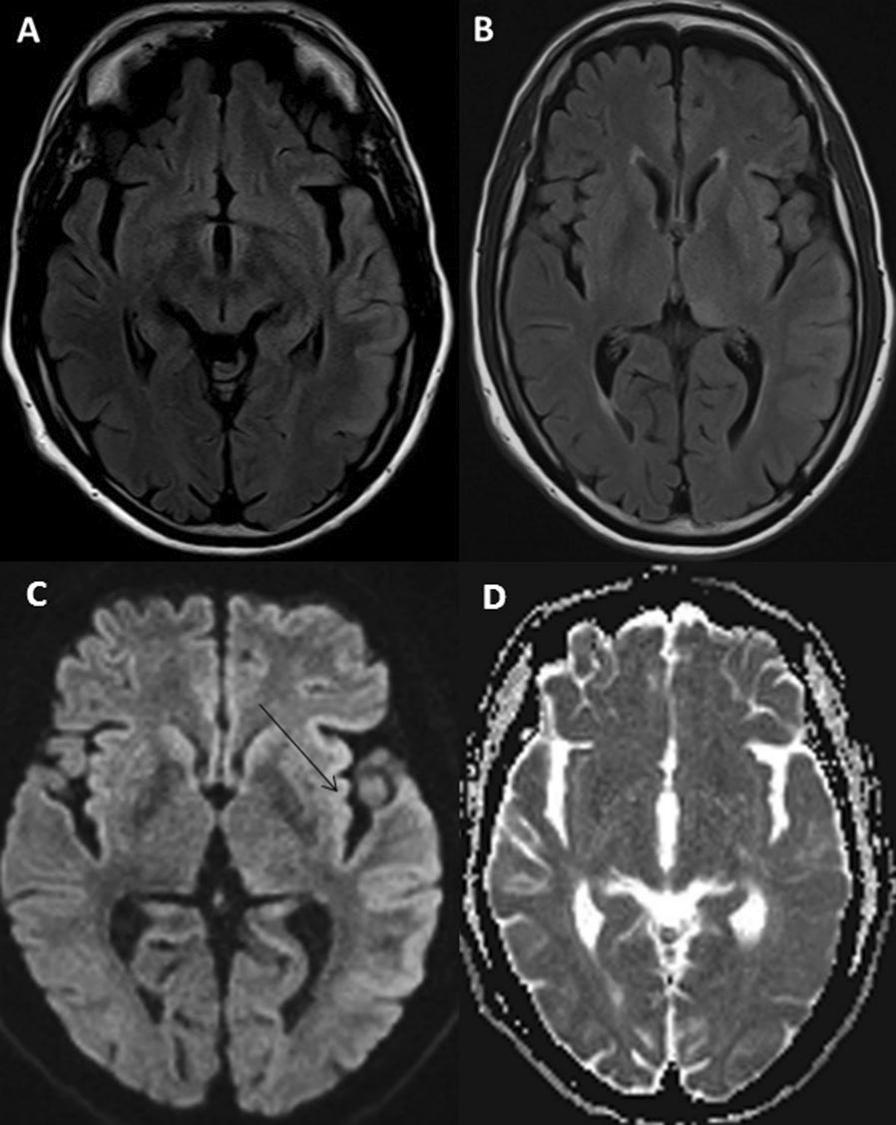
A and B Magnetic resonance imaging T2-weighted image shows hyperintensities in left temporal lobe, insula and left thalamus. C and D Diffusion restriction with normal apparent diffusion coefficient. Black arrow shows “insular ribboning”
